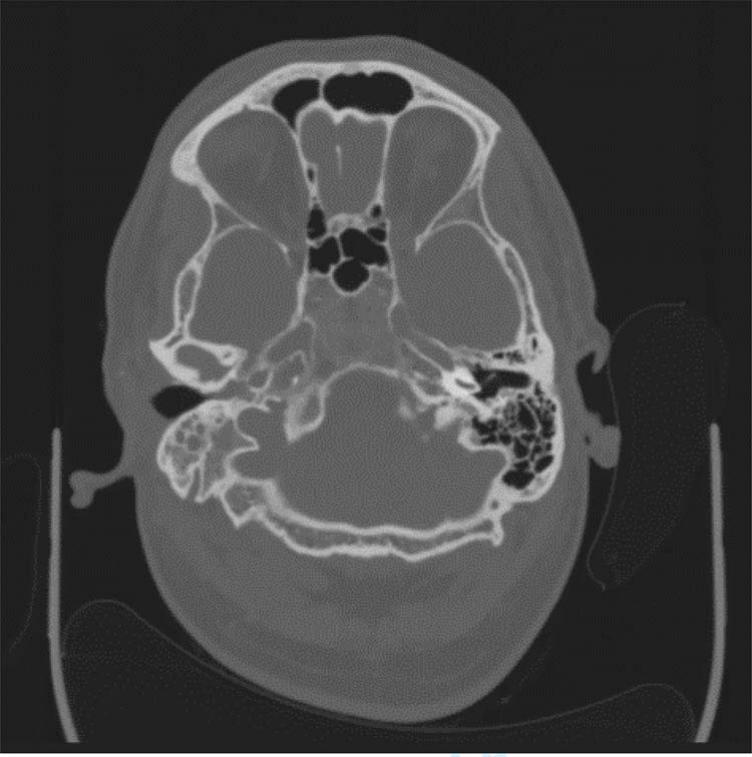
Axial CT image slice showing opacification of the right middle ear cavity and mastoid air cells.
